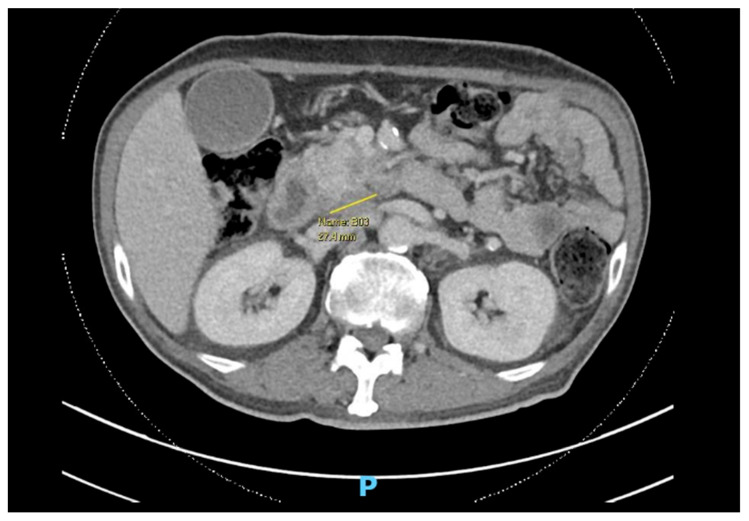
Computed Tomography (CT) scan showing axial cut of pancreatic malignancy.
